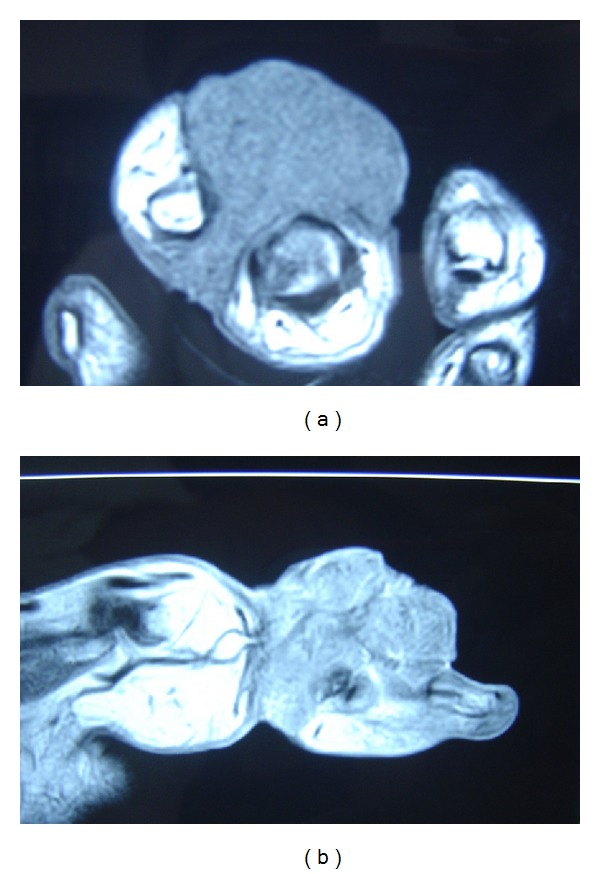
Preoperative MRI of the left hand shows an infiltrating soft-tissue mass with suspicion of malignancy (histiocytoma or fibrosarcoma) without osseous involvement.
